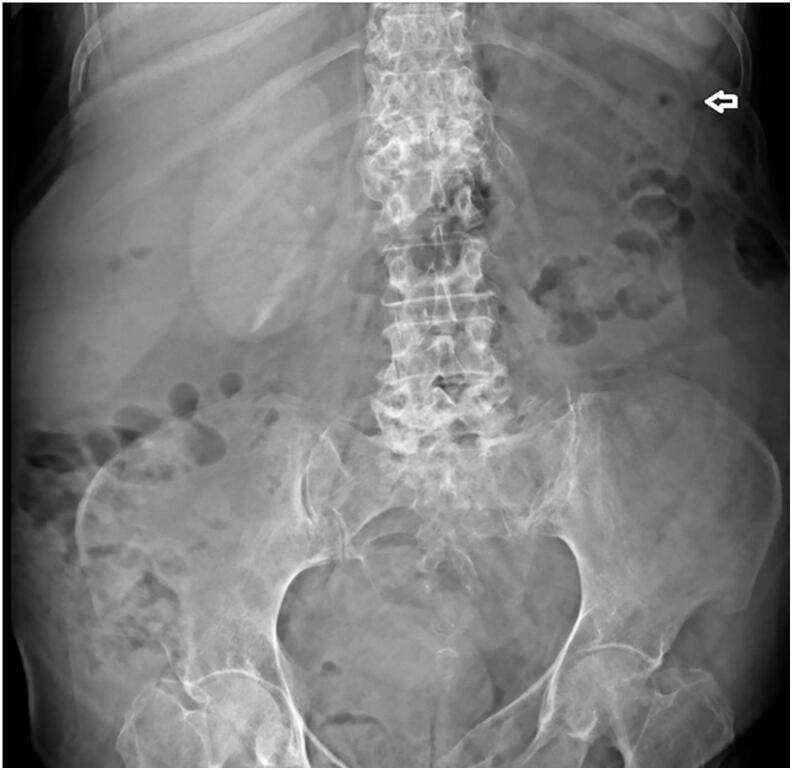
Plain abdominal radiograph (anteroposterior): pressure effect on and downward displacement of left kidney by a well- defined low-density mass is seen.
